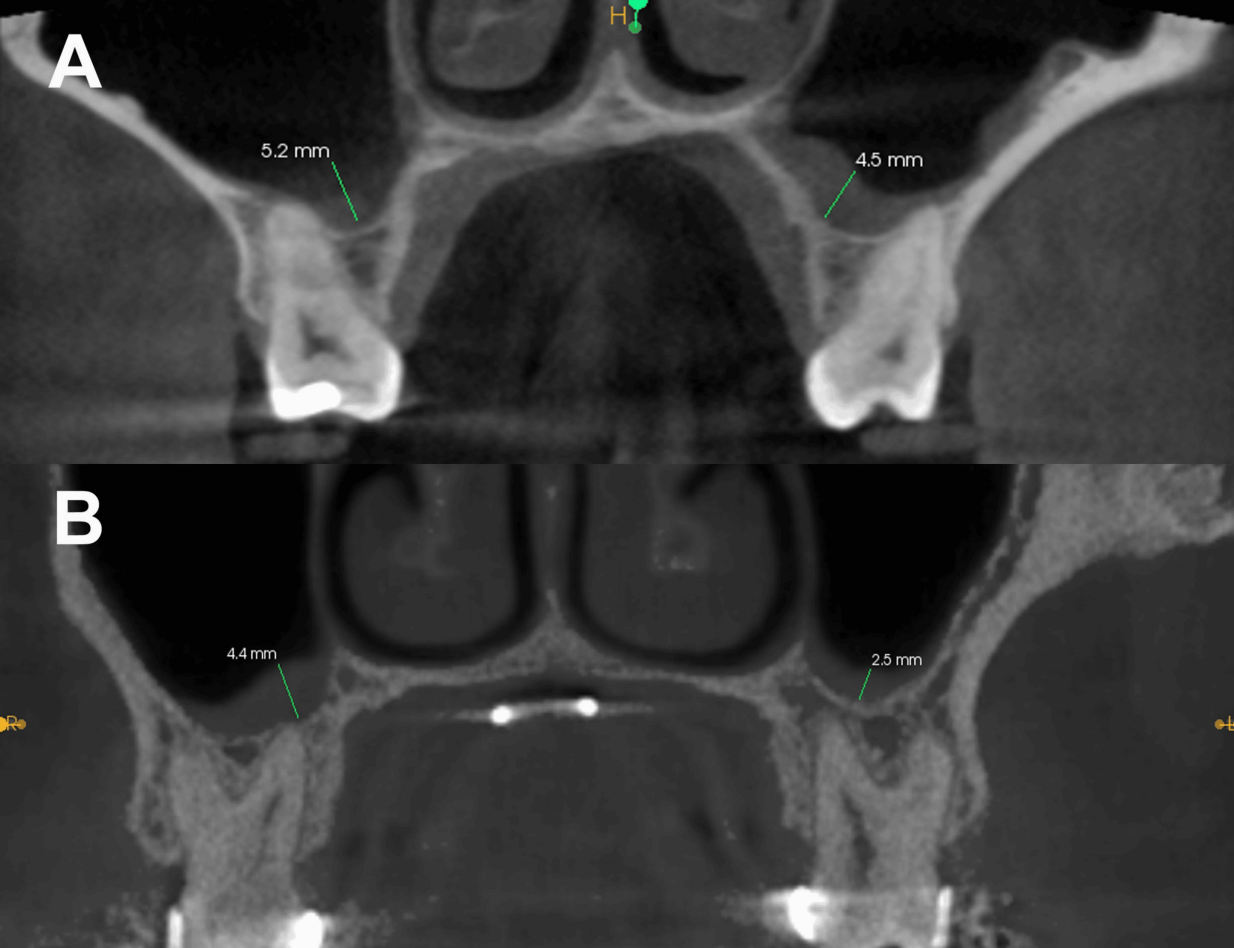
Maxillary sinus floor lining thickness in mm A: Smokers; B: Non-smokers This figure is taken from a CBCT scan of a patient included in the study.
